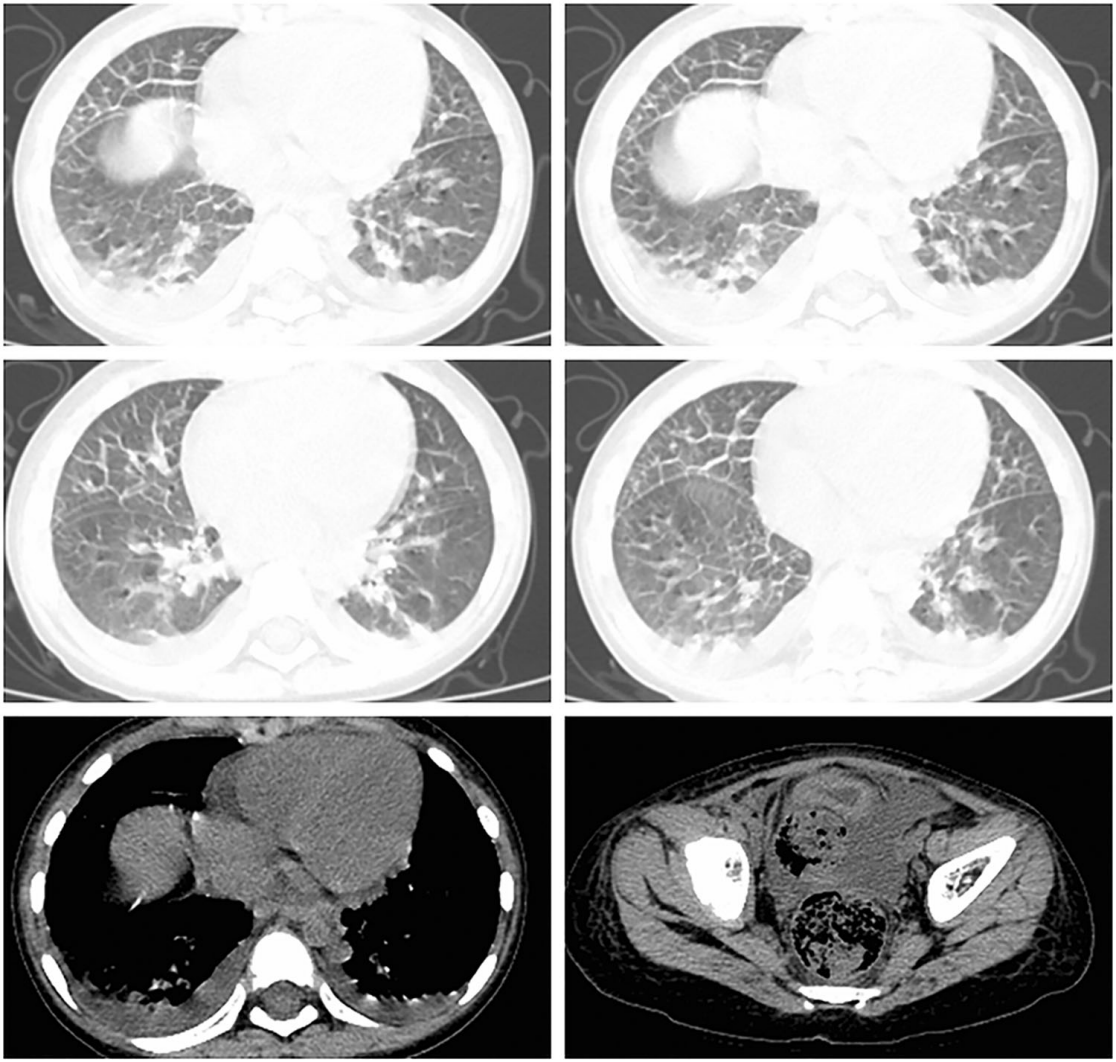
The chest and abdominal CT after admission in the pediatric patient, results are as follows: 1. infectious lesions in the lower lobes of the bilateral lungs, bilateral pleural effusion; 2. considerable stagnation of the contents of the colorectal cavity; 3. a little flocculent osmosis in the retroperitoneum, and the possibility of infectious diseases could not be excluded; 4. pelvic effusion. CT, computed tomography.
